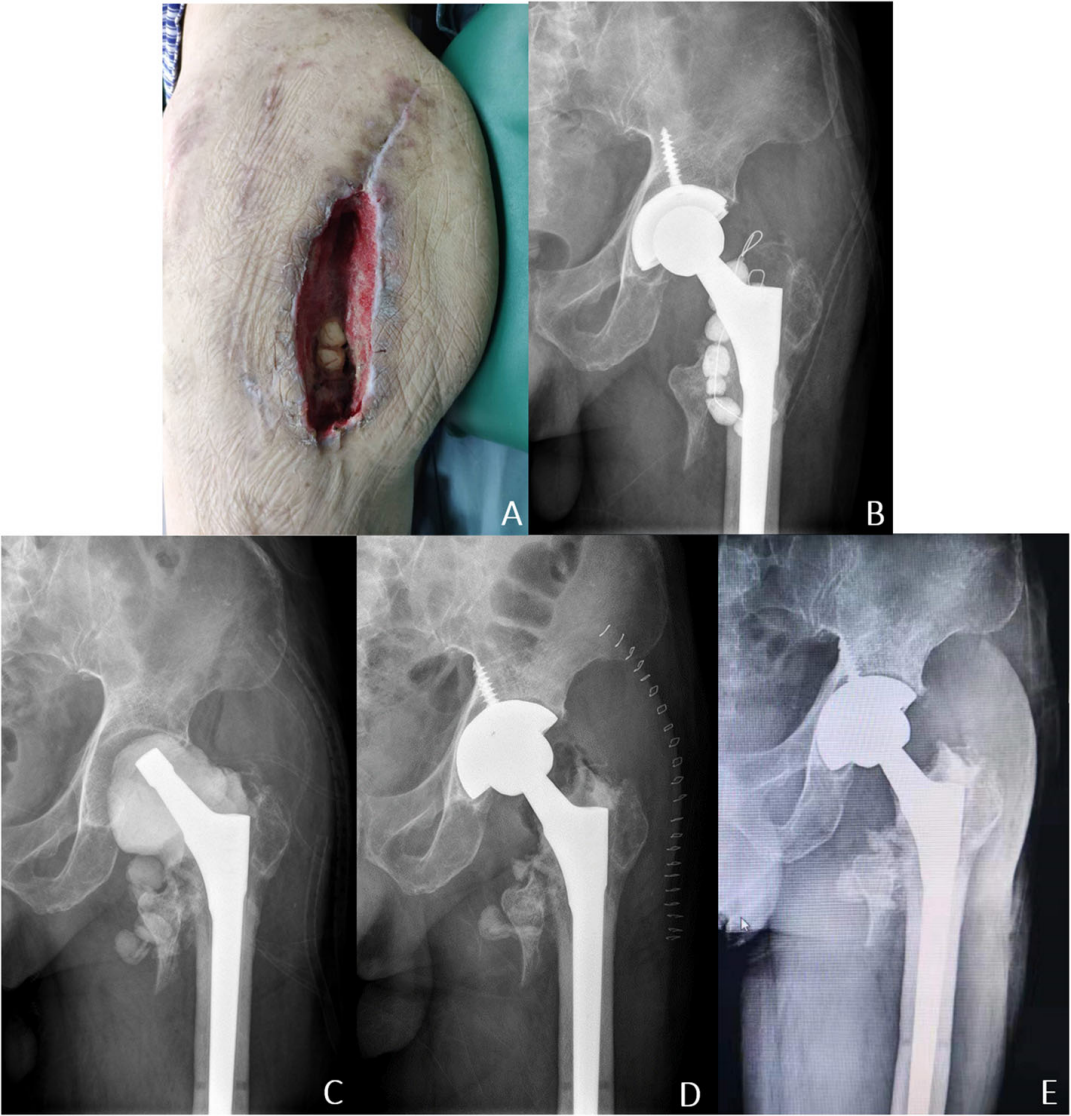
Left hip anteroposterior radiograph of patient 26. This patient who had undergone left THA half year previously was unfortunately infected. He underwent debridement and antibiotic-loaded cement implantation at the local hospital. (A) Photo taken when admission showed noticeable infection and massive soft tissue defects. (B) The X-ray showed no evidence of loosening of the acetabular cup and the femoral stem. (C) The acetabular cup was removed and a cement spacer was inserted in the first-stage operation. (D) At the second-stage operation, the spacer was removed, and the new implants were inserted. (E) There was no radiographic sign of implant loosening during the follow-up
